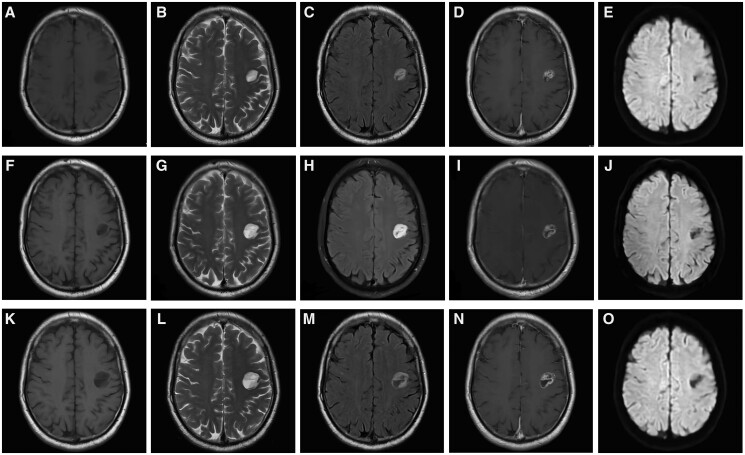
Imaging features of the myxoid glioneuronal tumour. Axial MRI showed a well-circumscribed cystic-solid lesion in the white matter area of the left frontal lobe. T1WI showed inhomogeneous low signal (A, F, K). T2WI showed inhomogeneous high signal (B, G, L). T2FLAIR shows significant high signal at the edge of the lesion (C, H, M). Contrast administration showed inhomogeneous and obvious ring-like contrast enhancement (D, I, N). DWI showed iso-low signal, with no obvious diffusion restriction (E, J, O). The lesion showed progressive enlargement during the three follow-up visits in October 2021 (A-E), February 2022 (F-J), and June 2022 (K-O). DWI, diffusion weighted imaging; MRI, magnetic resonance imaging; T1WI, T1-weighted imaging; T2WI, T2-weighted imaging.
